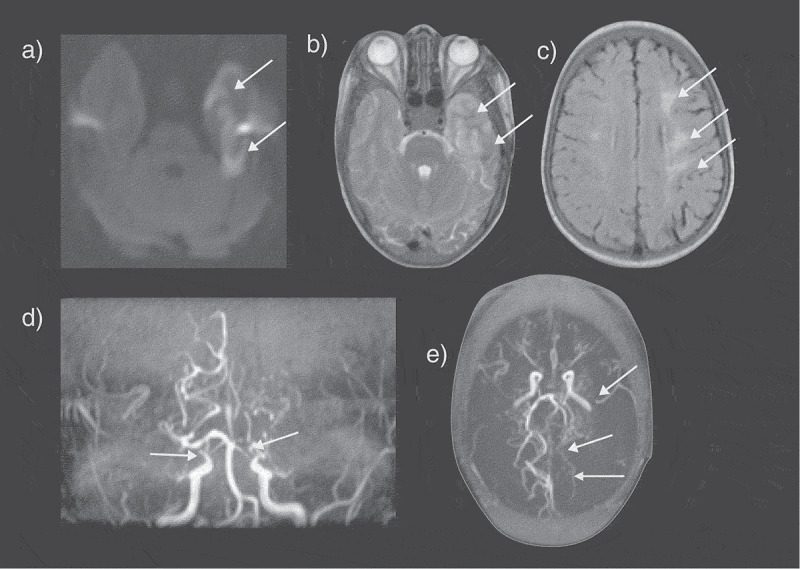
An SCD patient presented with seizures and a right sided neurological deficit (B) Axial T2-weighted images shows an acute left temporal lobe infarct in the left middle cerebral artery (MCA) territory with (A) restricted diffusion on the apparent diffusion map (ADC) map (arrows). (C) The FLAIR sequence revealed extensive mature ischemic changes within the subcortical, deep and periventricular white matter of the centrum semiovale, more marked on the left (arrows). (D) MRA revealed bilateral occluded terminal internal carotid arteries (ICA) and multiple moyamoya and pial collaterals (arrows). (E) The left posterior cerebral artery (PCA) is narrowed with distal pial vessels visible (arrows). FLAIR = fluid attenuated inversion recovery
